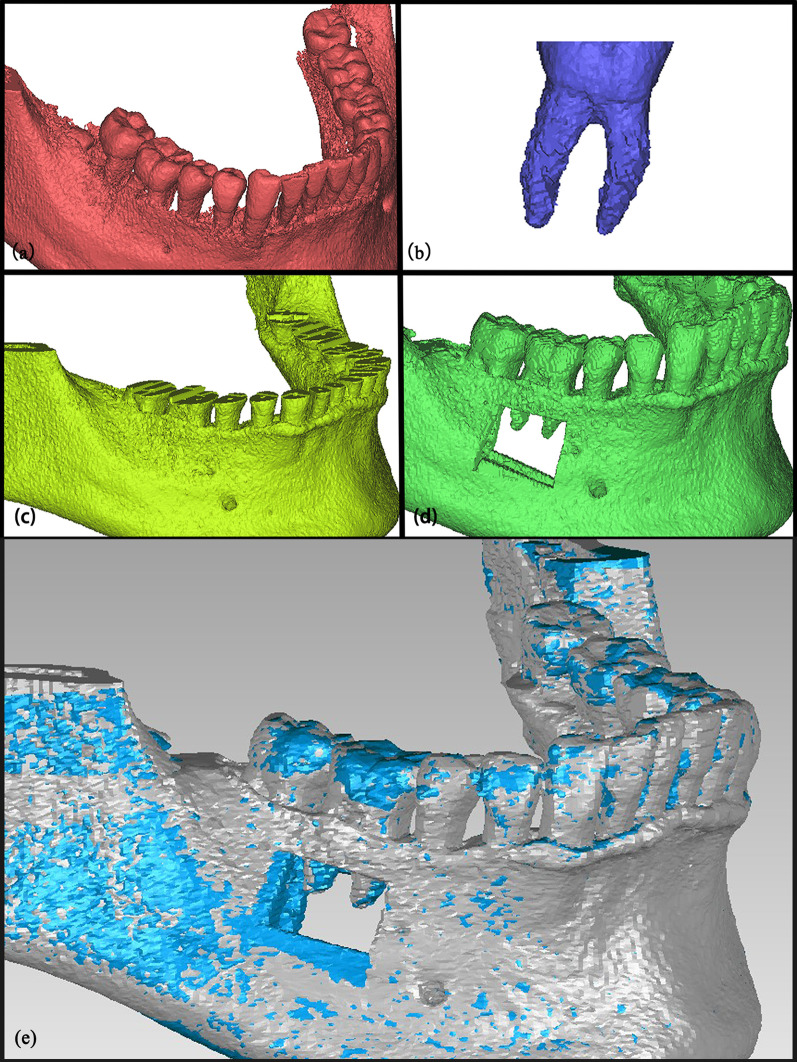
Processing in extracting CBCT 3D model information. a Crown: extracting 3D model under the experience threshold of crown; b Root: extracting 3D model under experience threshold of root with removing part of cusps; c Alveolar bone: extracting 3D model under the experience threshold of alveolar bone with removing the crown part; d Model for measurement after uniting; e The alignment of preoperative and postoperative CBCT images
